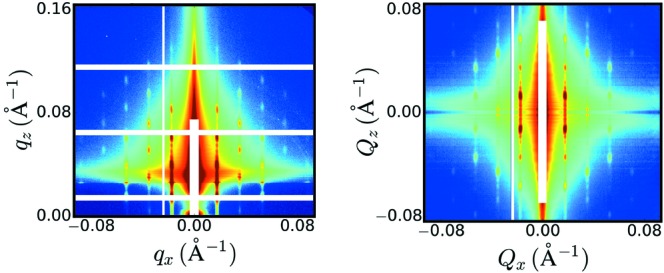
Correcting X-ray vision of thin films: the unwarping algorithm by Liu and Yager takes experimental GISAXS data (left), and generates a corresponding SAXS pattern (right). [Figure adapted from Liu & Yager (2018 ▸) with permission.]
